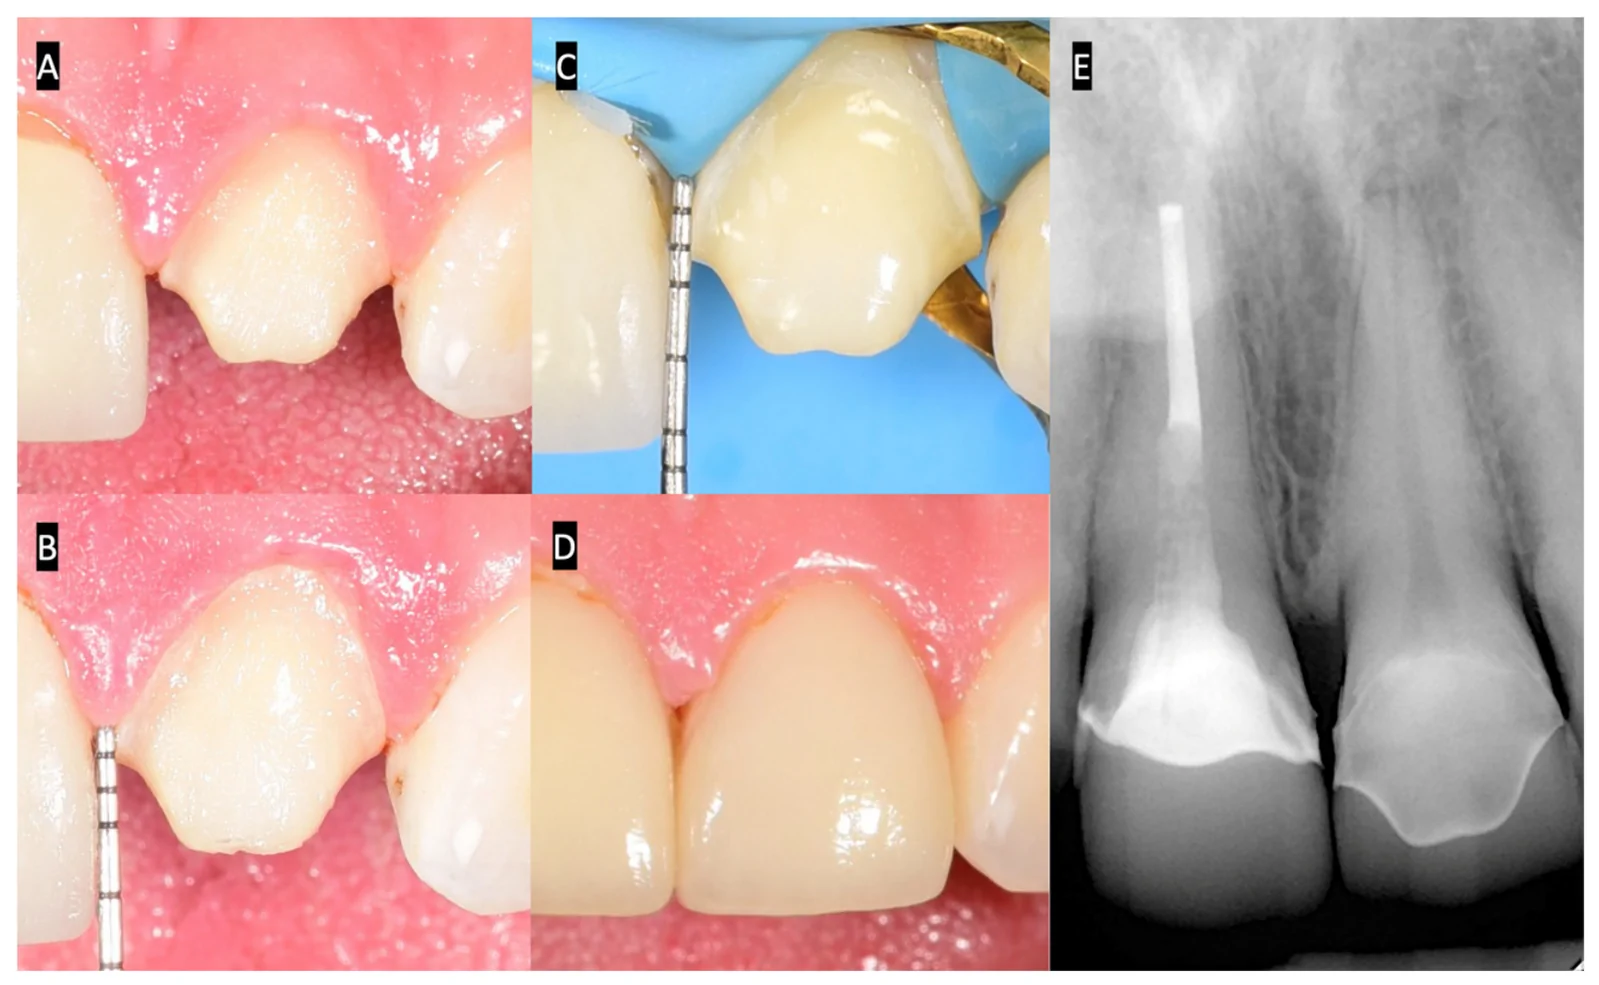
Zone 1 clinical scenario. (A) Initial clinical situation; (B) evaluation of margin depth with a periodontal probe; (C) treatment under dental dam isolation, showing the new margin depth evaluated with a periodontal probe; (D) final restoration; and (E) final radiograph after crown cementation.
